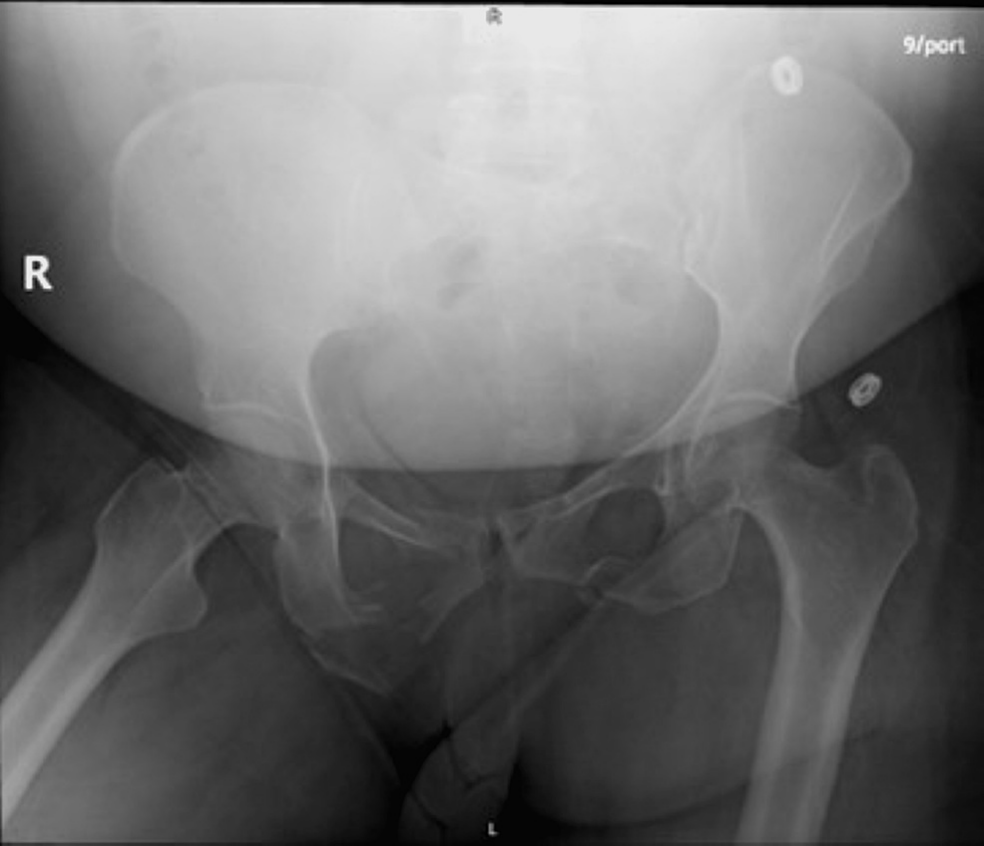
Radiograph showing resultant post-motor vehicle accident right superior and inferior pubic rami fracture with no extension to the sacrum, as well as left superior and inferior pubic rami with extension to the sacrum.
